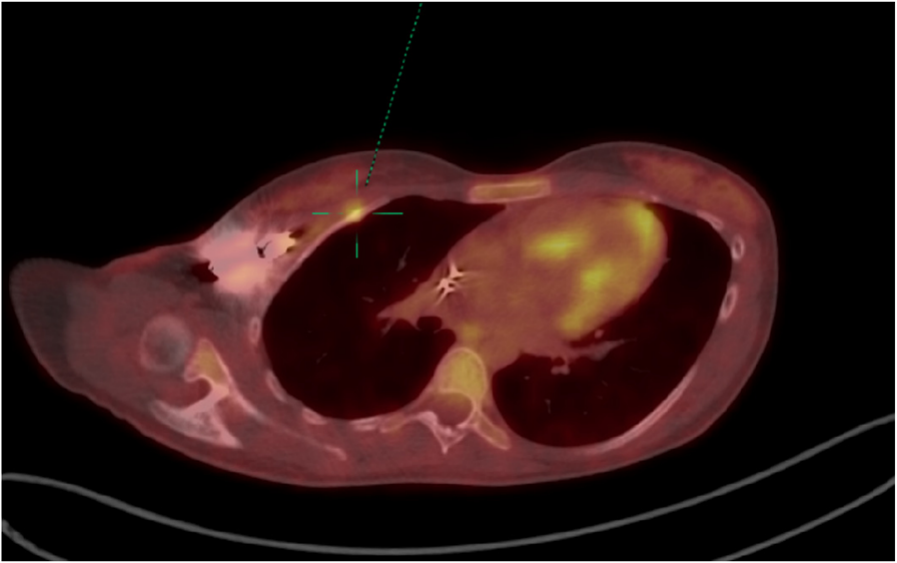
Fusion positron emission tomography CT (PET CT) image in oblique axial plane depicting pacemaker generator and metabolic activity (dotted line) in the healing fracture in the anterolateral part of the second right rib
